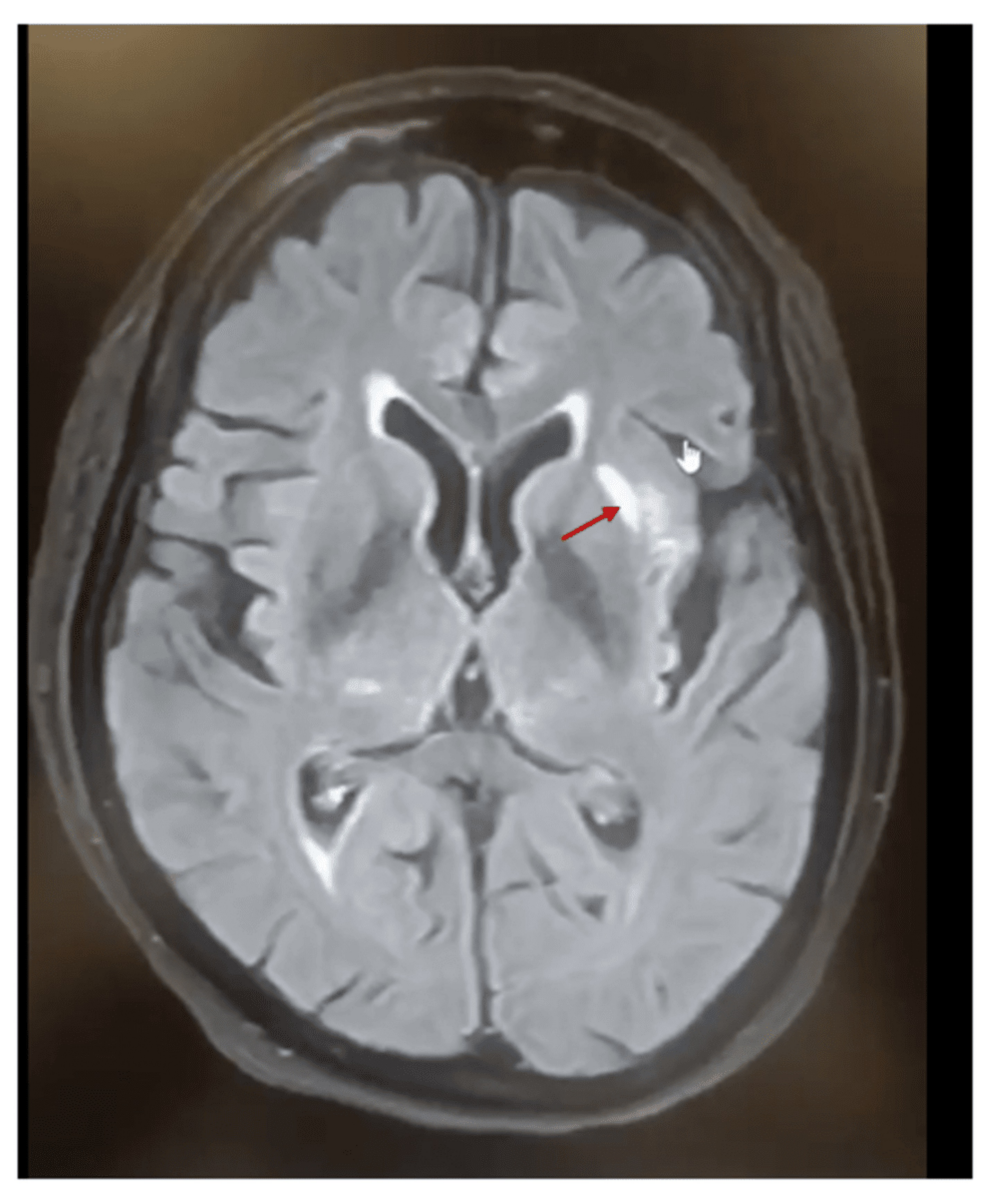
Magnetic resonance imaging confirming left non-hemorrhagic lacunar infarct in the frontal lobe.
